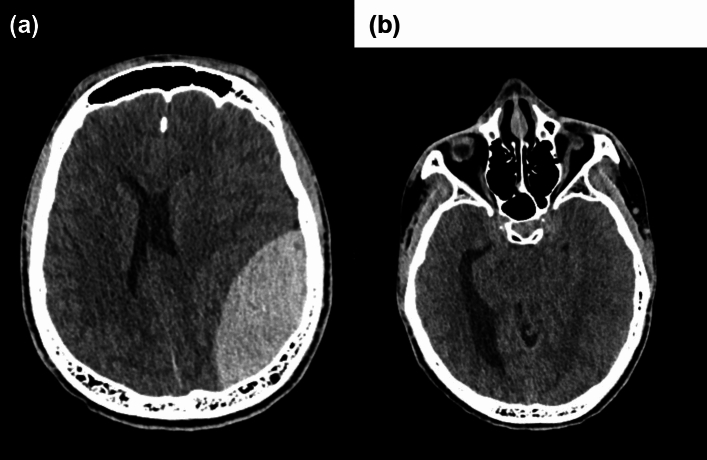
a and b 16-year-old player presented with deteriorating level of consciousness with a GCS of 7/15 at initial presentation. Axial CT imaging demonstrated a large left extradural haematoma (41 mm in depth), 8 mm midline shift, subfalcine and uncal herniation, and contralateral entrapment hydrocephalus
